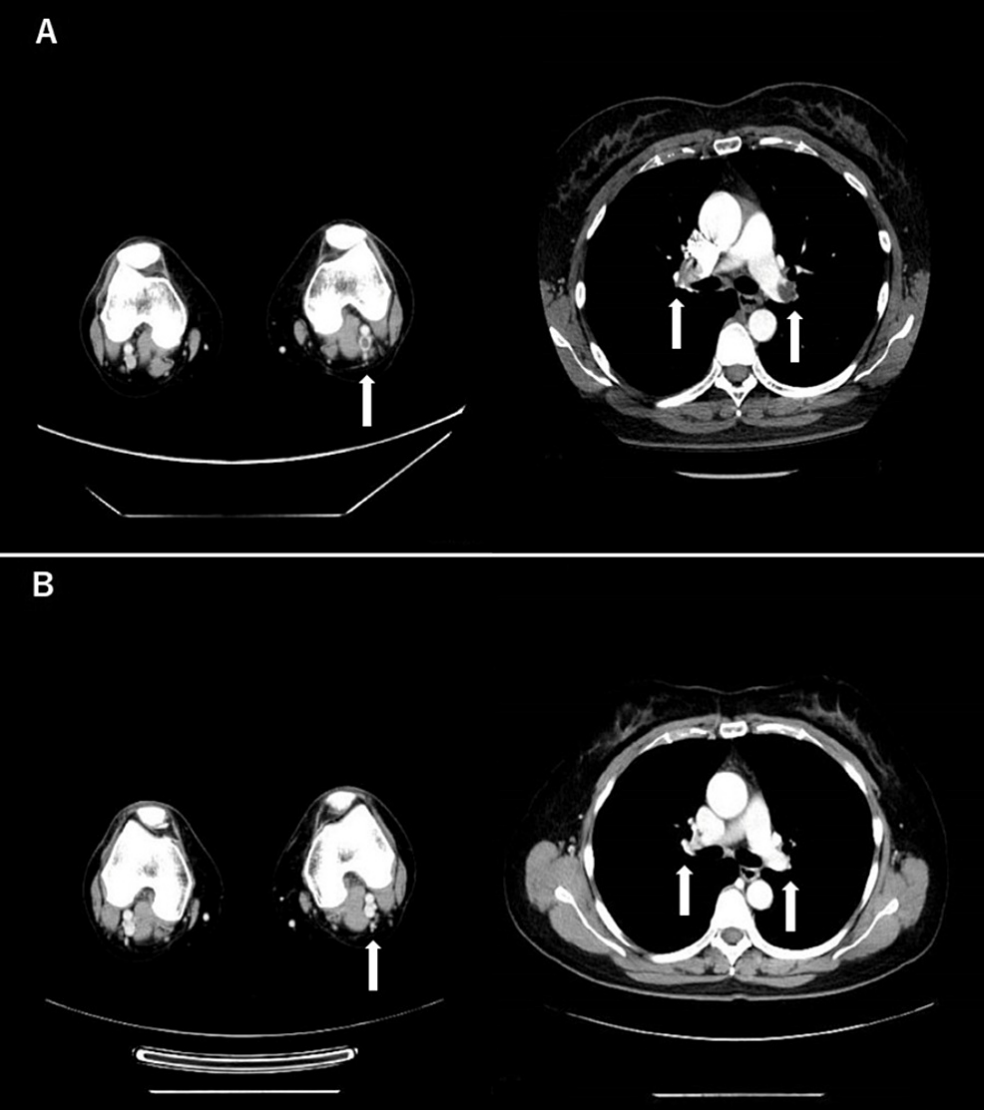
Computed tomography images taken on days 48 and 152 (day one was the day of the first COVID-19 vaccination) for a woman with deep vein thrombosis and pulmonary embolism after the second inoculation A. Contrast-enhanced CT shows thrombi in the bilateral pulmonary artery trunk and left popliteal vein on day 48. B. Contrast-enhanced CT on day 152 confirms the disappearance of the thrombi.
